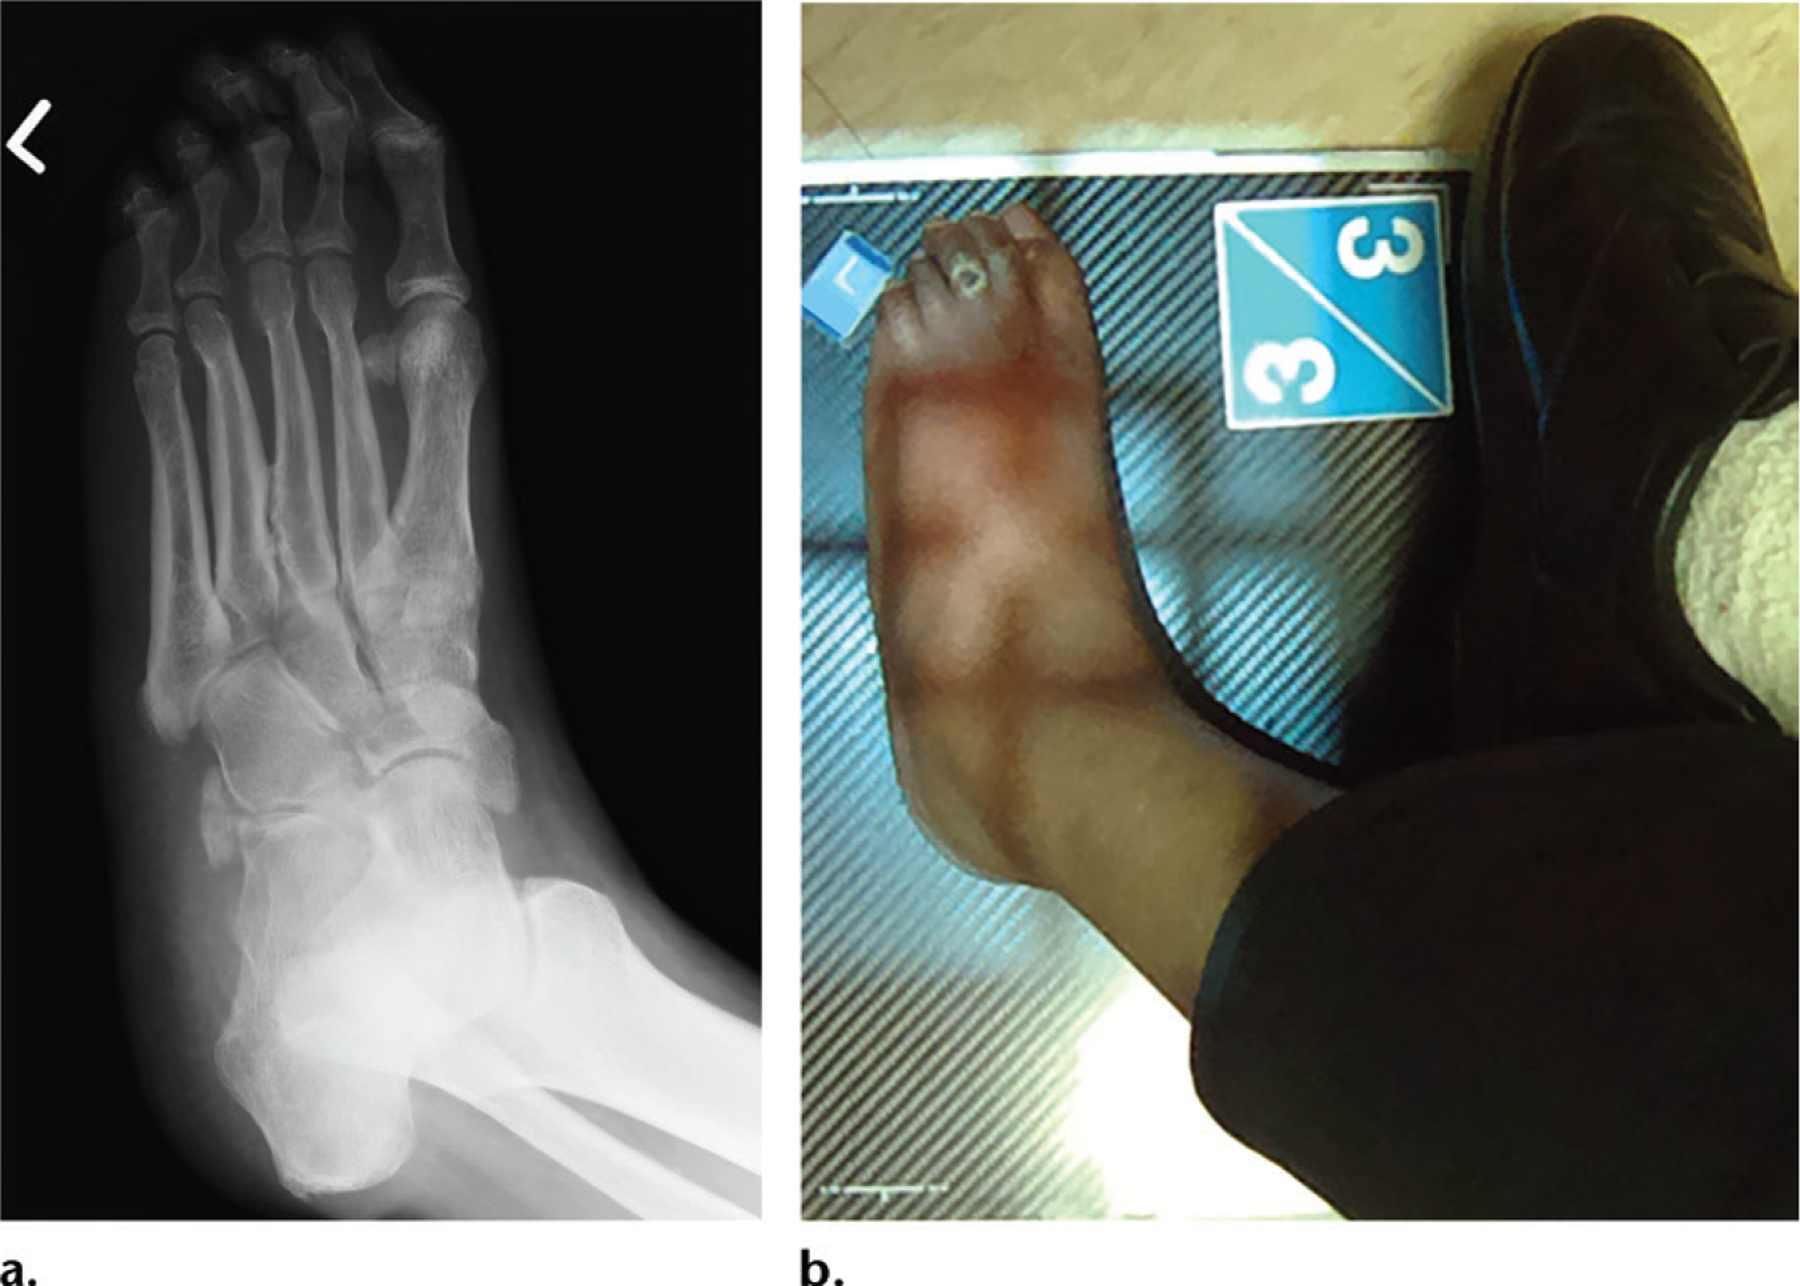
(a) Oblique radiograph shows the left foot in a 46-year-old man who underwent imaging for possible osteomyelitis related to a diabetic foot ulcer. The interpretation can be substantially accelerated if there is an indication of the location of the ulcer. (b) Coned-down photograph shows the ulcer on the third toe of the left foot, which helps focus the radiographic evaluation.
